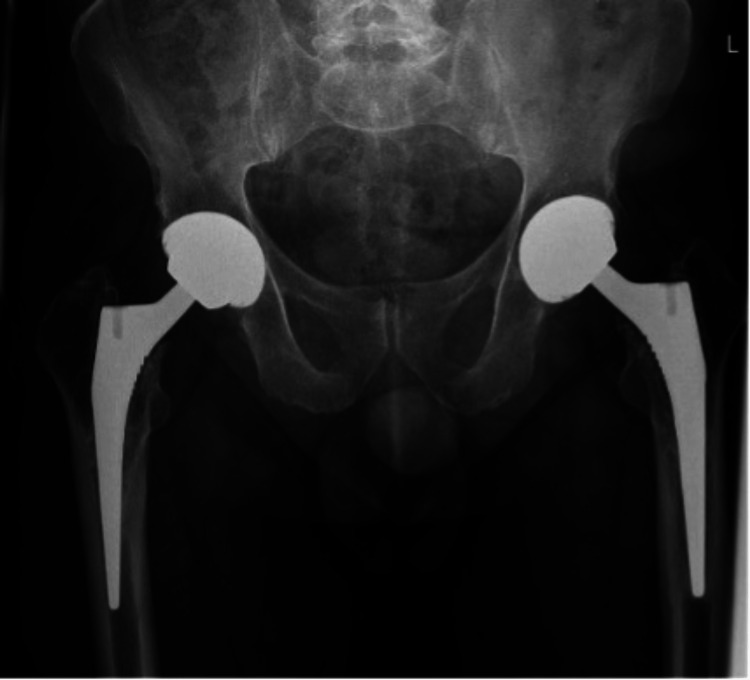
X-ray (anteroposterior view) of bilateral hip joints taken one year back showing bilateral uncemented implants in situ
